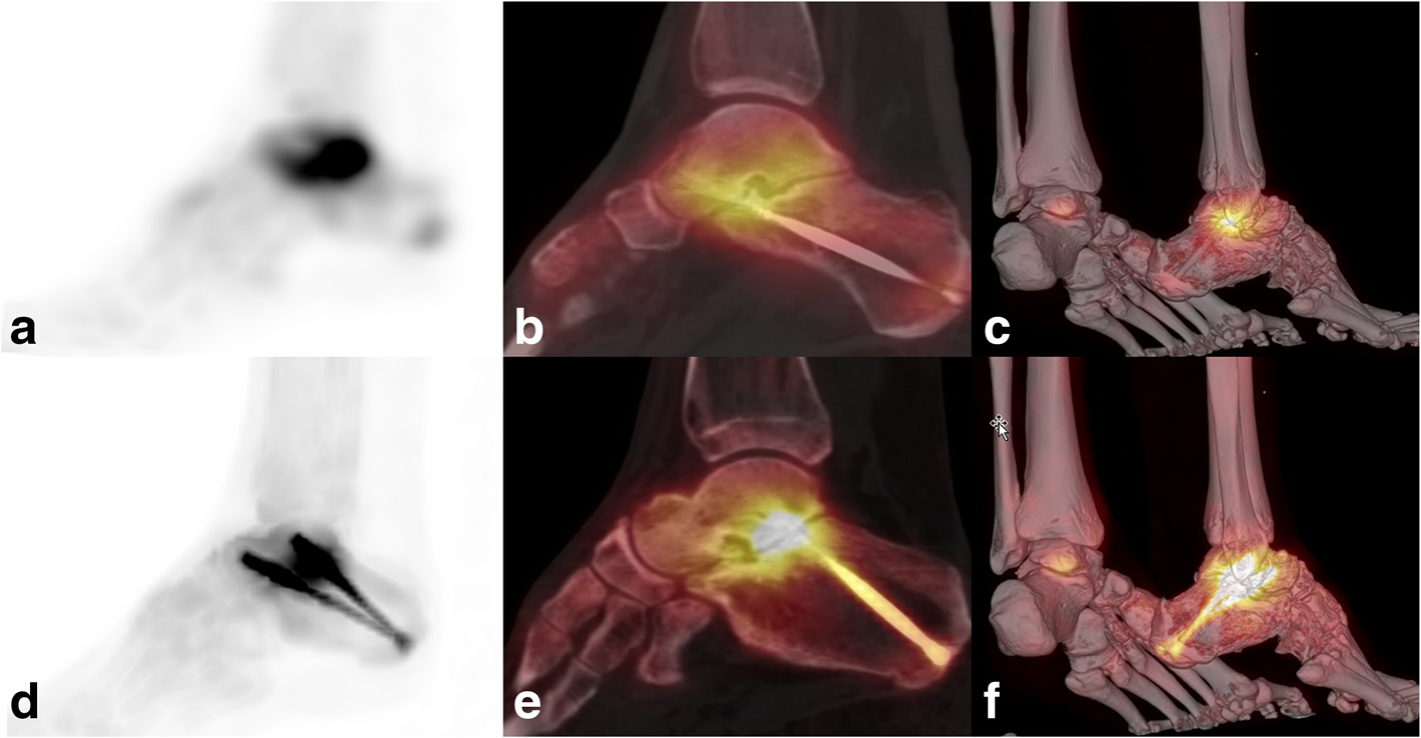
xSPECT Bone “shining metal artefact” in the foot. SPECT/CT images of a 35-year-old female patient with pain in the ankle 17 months after arthrodesis of the right lower ankle joint. MIP image with xSPECT Bone reconstruction (d), fused sagittal SPECT/CT (e) and fused 3D reconstruction (f) show high “pseudouptake” of the screws and true increased uptake in the non-union. Corresponding images (a–c) with conventional Flash3D reconstruction show no such uptake of the radiotracer around the screws and no morphologic signs of loosening with maintained true uptake of the non-union. Re-arthrodesis was performed as a consequence of the SPECT/CT results
